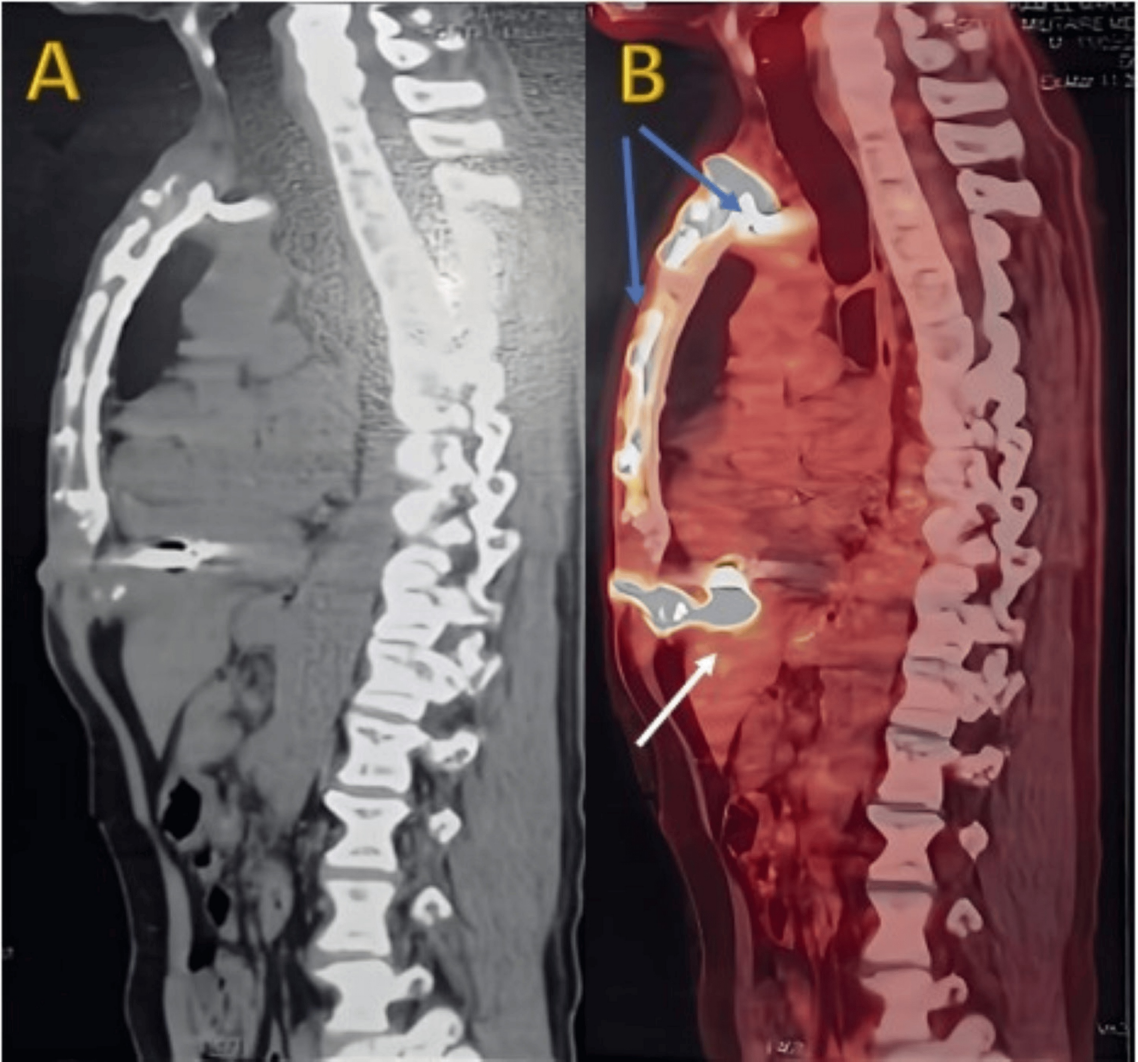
(18F)-FDG PET scan revealing pathological hypermetabolism and associated findings in cervicothoracoabdominal sections On PET scan with (18F)-FDG imaging in sagittal cervicothoracoabdominal sections, prior to tracer injection (A), retrosternal material is observed extending into the left subclavian region (proximal end of the ICD lead), projecting over the subclavian vein and left innominate vein. Additionally, hyperdense material (right ventricular lead tip) generates artifacts at the apex of the right ventricle. One hour after (18F)-FDG tracer injection (B), intense pathological hypermetabolism is noted in the left supraclavicular region along a muscular trajectory and retrosternally, extending into the left subclavian area near the innominate vein, likely of infectious origin. This is associated with linear pathological hypermetabolism involving the manubrium and sternal body (blue arrows), extending superiorly to a pathological median suprasternal cutaneous hypermetabolism and inferiorly to hypermetabolism below the sternal notch. It further extends intrathoracically at the level of the right ventricle, adjacent to the residual material (white arrow). FDG: fluorodeoxyglucose; ICD: implantable cardioverter defibrillator
